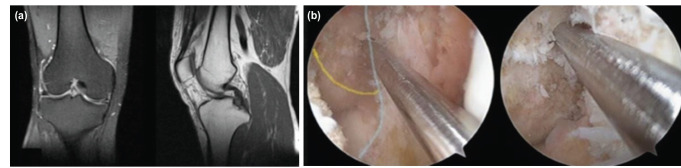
The 32-year-old male patient has ACL injury in the right knee, (a) Sagittal and axial section MR image, (b) Placement of the guidewire, the appearance of the distance to the inferior and posterior cortex, preventing PCL from the visualisation of the posterior cortex (blue line).
